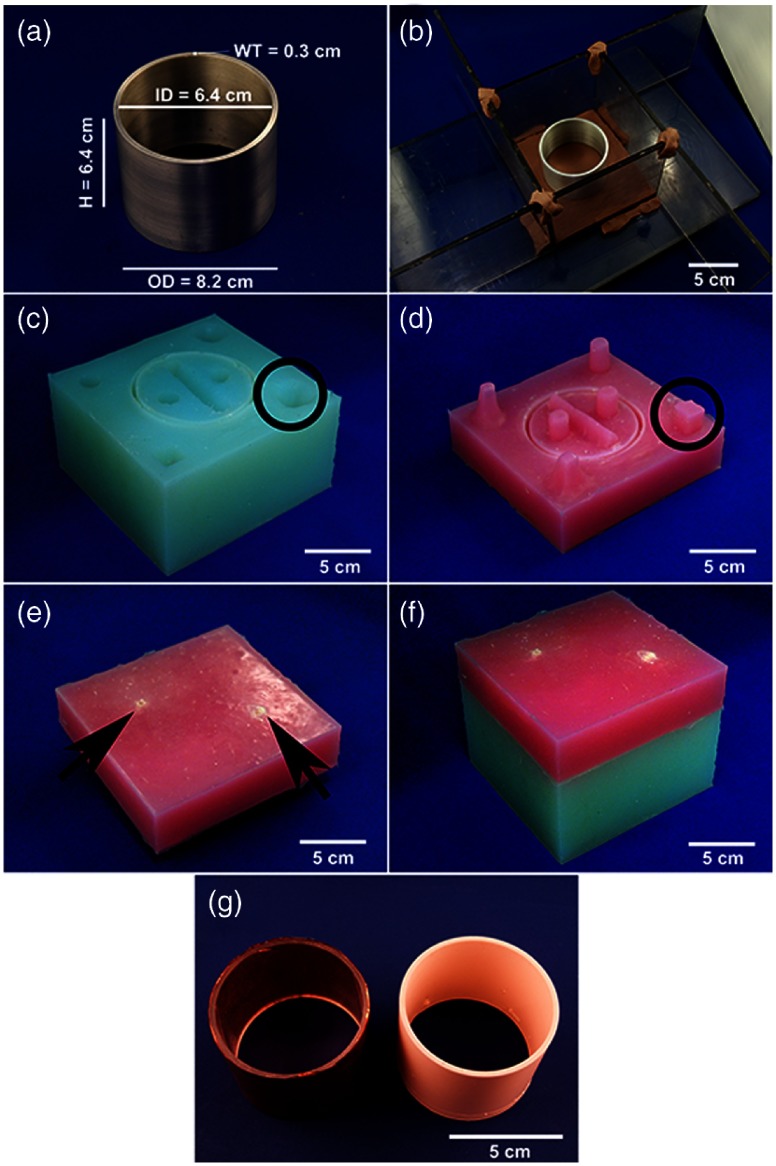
Process implemented to fabricate a custom silicone mold used to cast custom resin x-ray filters. (a) Master machined Al filter. (b) Assembled box, comprised of multiple acrylic pieces, to encompass the two-part silicone that is to be poured over the embedded Al filter within a layer of sulfur-free clay. (c) Extracted silicone mold representing the bottom-half of the silicone mold. Circle emphasizes one of the seven registration keys that were used to ensure accurate assembling of the silicone bottom- and top-half. (d) Silicone mold of the top-half. Circle represents the corresponding registration key from (c). (e) Top-half of the silicone mold flipped to demonstrate the fill and evacuation ports (arrows). (f) Assembled silicone mold. (g) Fabricated custom x-ray filters, the erbium-impregnated resin casted low-energy filter (right) and the copper foil wrapped around acrylic core high-energy filter (left).
